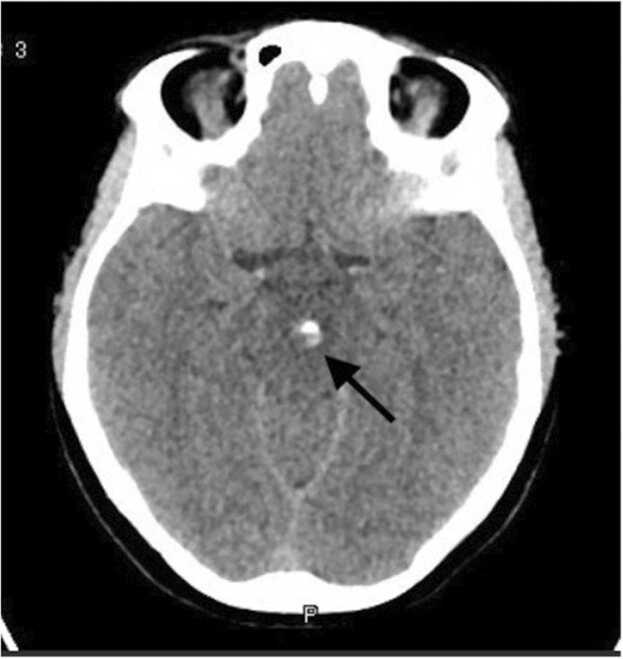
Cross-sectional view of CT brain showing a focal hyperdense calcified lesion measuring 6 mm in the midline portion of the midbrain (black arrow).
